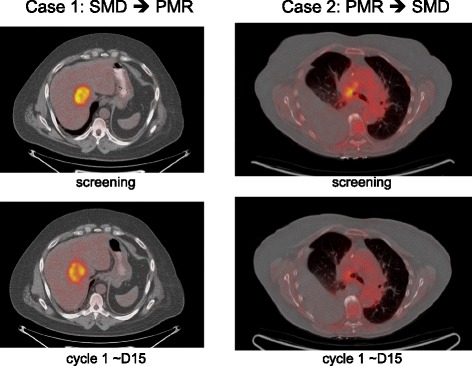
Example fused axial PET/CT images for patients with metastatic NSCLC whose response classification was changed using our new criteria (compared to the EORTC criteria). Case 1 had a single liver lesion that showed a 20 % decrease in SUVmax after 2 weeks of treatment and was classified as SMD by EORTC criteria. This patient was reclassified by our scheme as PMR because the baseline lesion SUVmax was 19, resulting in a narrow confidence interval for spurious changes. Case 2 has a single upper lung lesion demonstrating a 33 % decrease in SUVmax that was classified as PMR using the EORTC criteria. This patient was reclassified to SMD using our criteria, as a result of a low screening SUV value of 3.3. (These cases are represented in Fig. 3b as patients #4 and #22)
